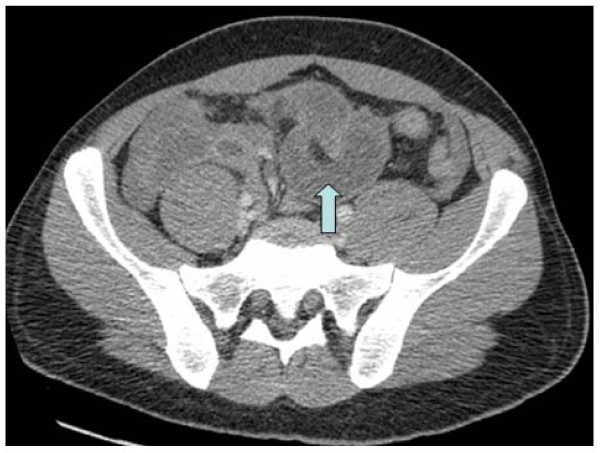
CT scan depicted the origin (*arrow key*) of the telescoping of the ileal segment.
